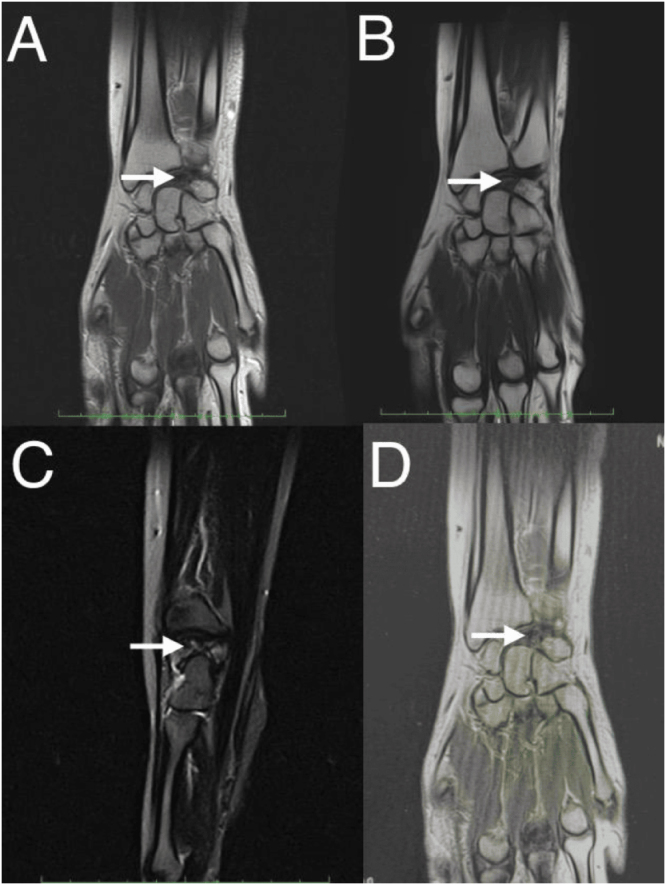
A: MRI of right wrist, coronal T1W sequence showing, relatively smaller sized lunate with hypointense signal (arrow). B: MRI of right wrist, coronal T2W sequence showing hypointense signals from lunate bone (arrow). C: MRI of right wrist, sagittal T1W sequence with fat suppression, showing hypointense signals (arrow). D: MRI of right wrist, coronal T1W sequence with gadolinium injection showing, hypointense signals without post contrast enhancement (arrow).
